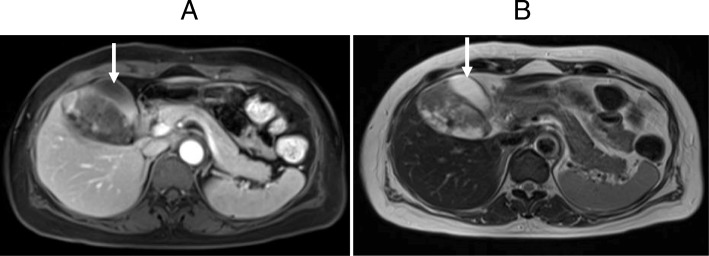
Abdominal magnetic resonance imaging found a tumor between the gallbladder (white arrow) and the liver. a T1 W1. b T2 W1
